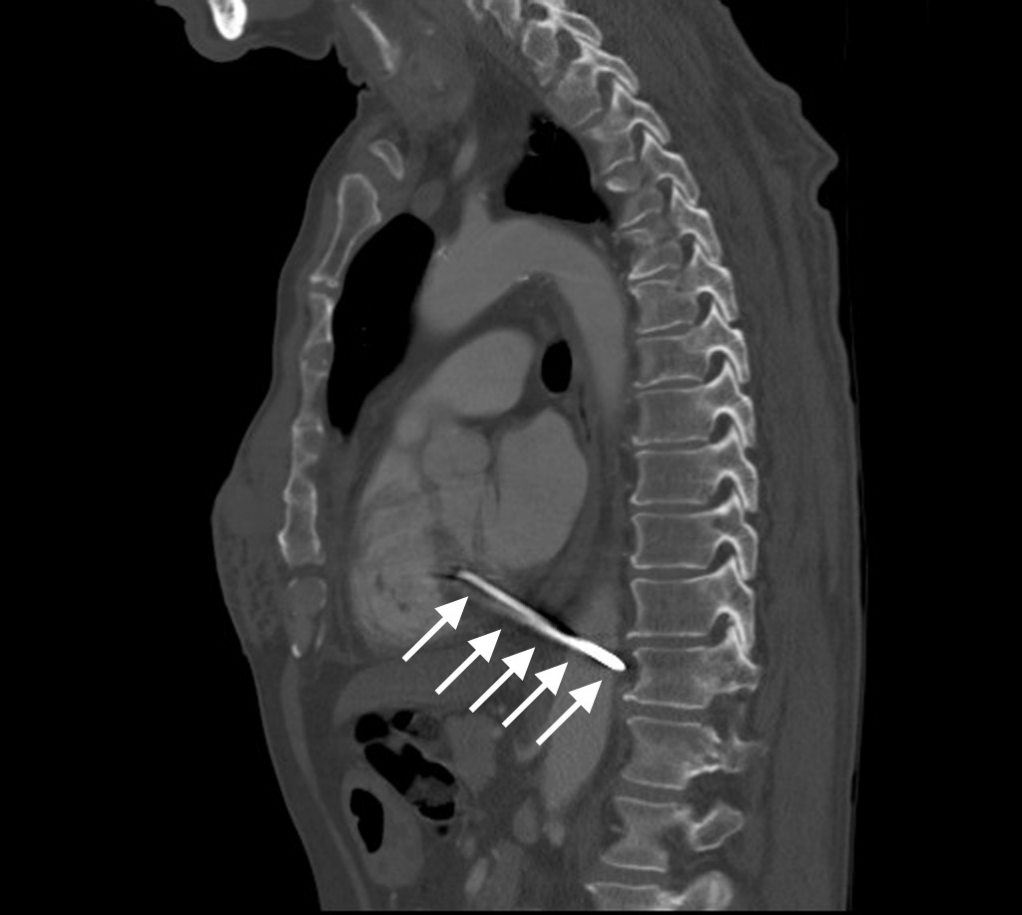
Sagittal reformatted contrast-enhanced CT Sagittal reformatted contrast-enhanced CT image showing longitudinal plane of the nail with the tip as previously described and the tail in the posterior wall of the right ventricle (arrows).
